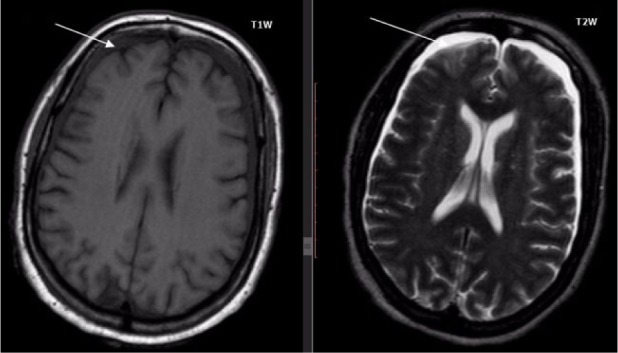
Brain MRI (T1W and T2W) showing bilaterally thin but widely spread subdural collection in the frontoparietal region.
